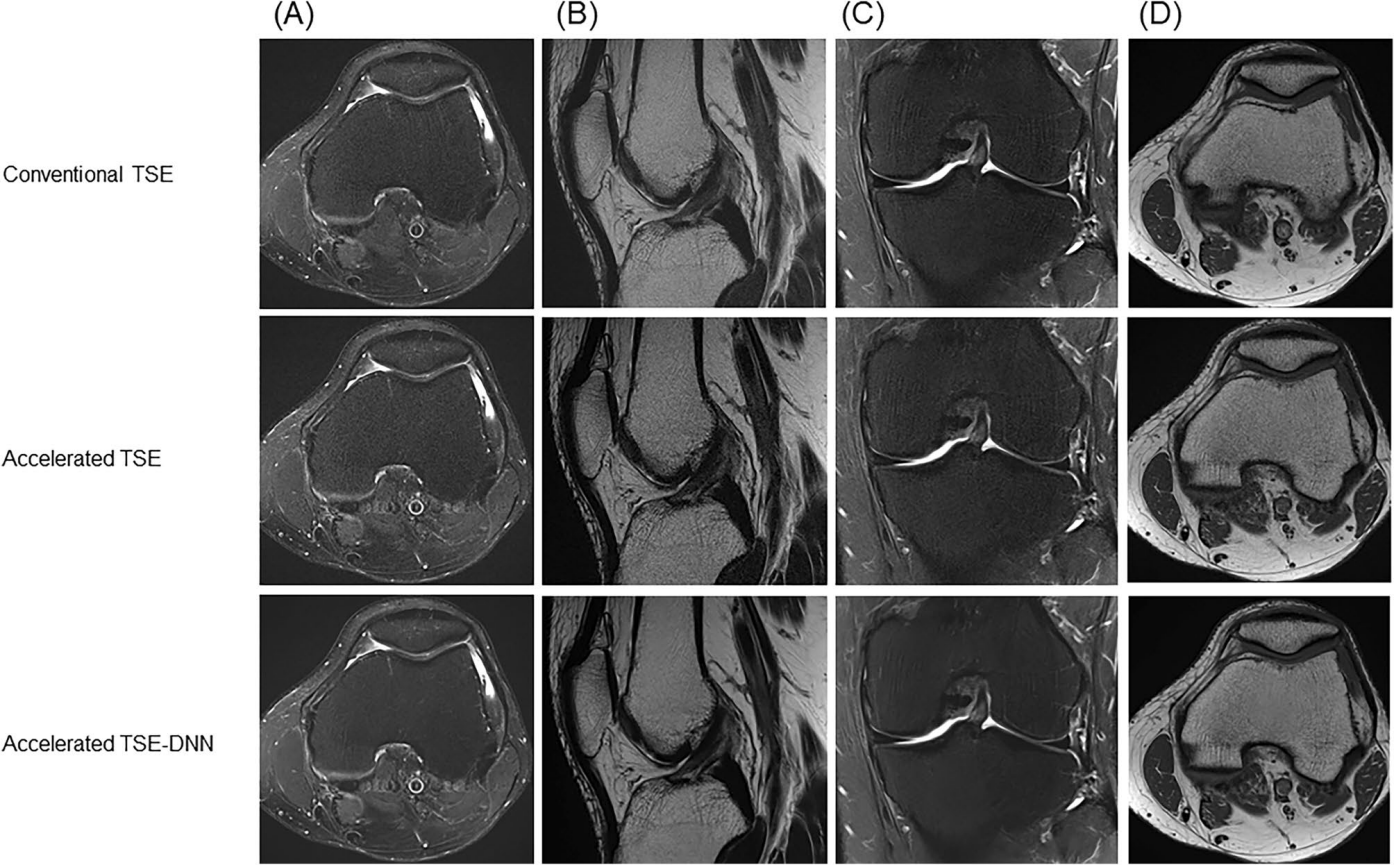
Conventional and reconstructed images of accelerated sequences: a 47-year-old male with knee pain (A–D). FSE-DNN shows comparable image quality with reduced scan time. The first row represents FSE-conventional images, the second row represents accelerated FSE sequences, and the third row represents DNN-reconstructed images of FSE-DNN. Each column represents the images reconstructed by axial fat-saturated T2-weighted image, sagittal T2-weighted image, coronal fat-saturated T2-weighted image, and axial T1-weighted image.
